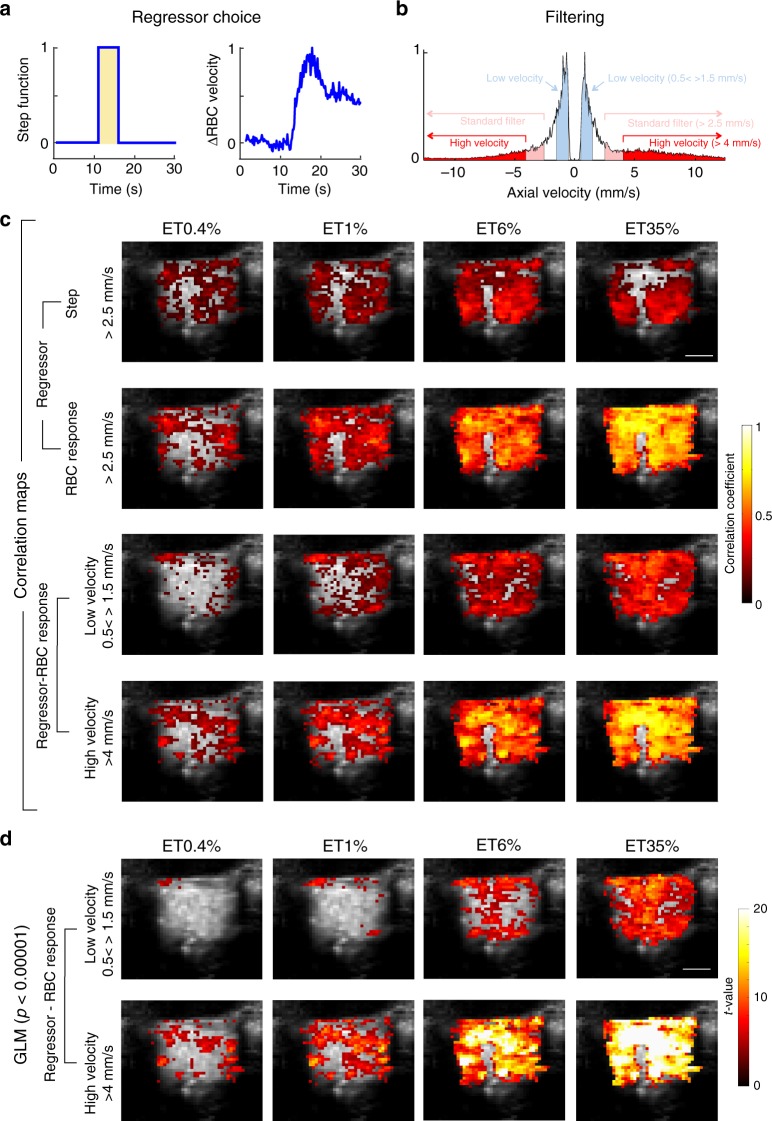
Mapping vascular activation with fUS. a Shows regressor used for building fUS activation maps: Left: step function; Right: capillary RBC velocity response (here at 35% ET). b Spectrum of Doppler frequencies converted to axial velocity (mm/s). Different filters were used for the analysis. Either a standard high-pass (HP) filter (reporting the CBV fraction flowing with an axial velocity > 2.5 mm), a higher HP filter reporting an axial velocity > 4 mm/s or a band-pass filter reporting an axial velocity between 0.5 and 1.5 mm/s). c Top two lines: comparison of the correlation maps obtained with the two regressors, using a standard HP filter. Using the RBC response as a regressor significantly improves the quality of the map, in particular in the dorsal OB. Bottom two lines: correlation maps for low axial velocities, i.e. comprising capillary responses differ from the large vessel maps (high axial velocities). d Activation maps of low and high axial velocity vessels using the general linear model with the RBC response as a regressor and a statistical threshold of (*p* < 0.0001)
